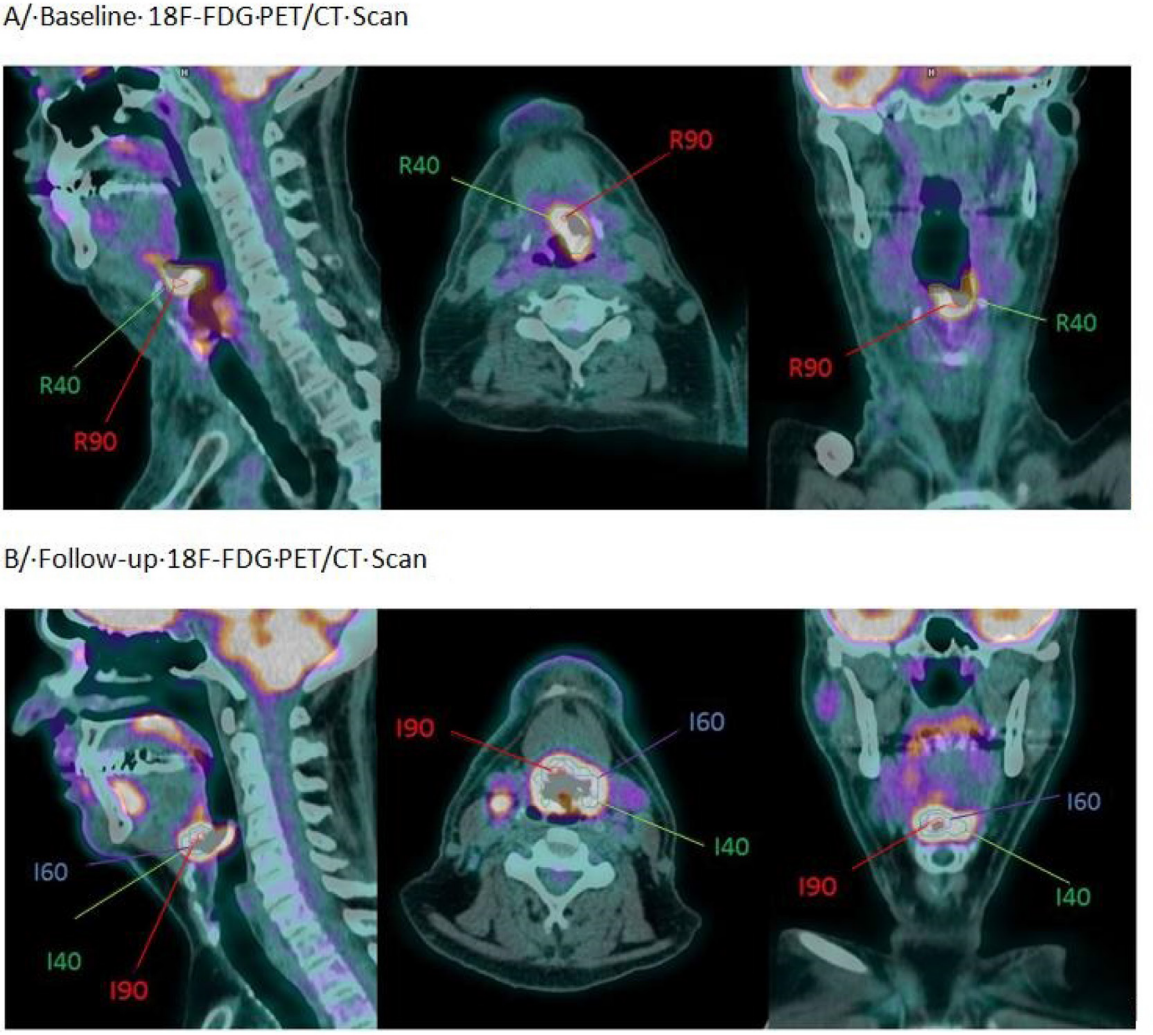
Example of an oropharyngeal cancer (stage T4N2M0) in a 54-year-old patient with residual disease (A) Baseline PET/CT Scan (pre-treatment). (B) Post-treatment PET/CT scan demonstrating a local recurrence.
